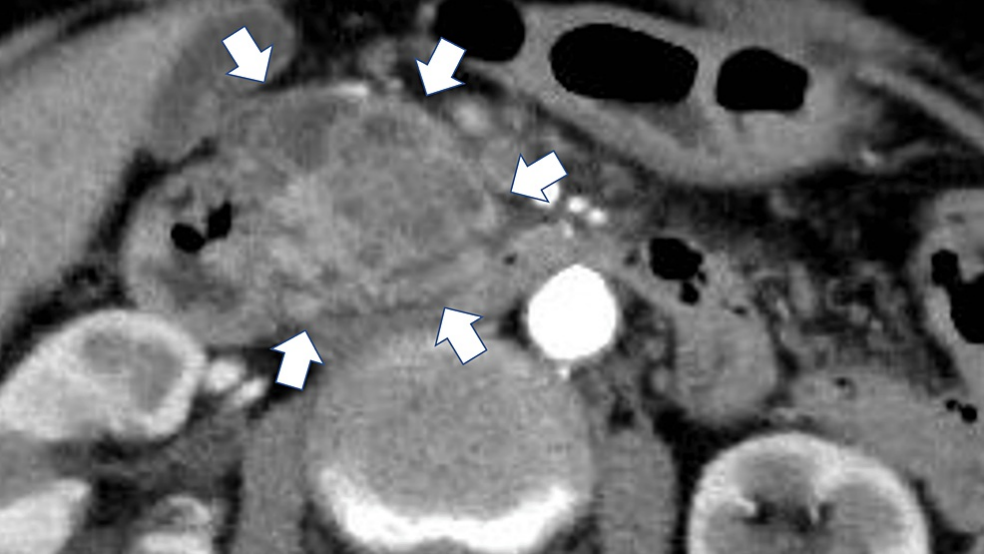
Dynamic CT showing a mass (d = 30mm) with few small cysts in the head of the pancreas (white arrows).
